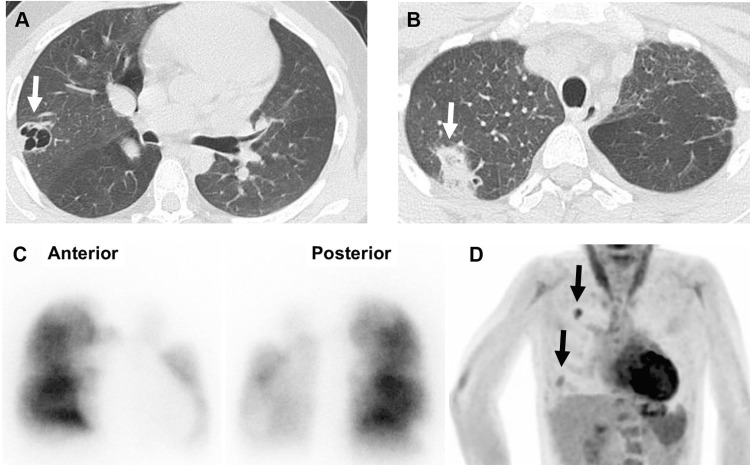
Images at admission A: Computed tomography (CT) indicated vesicles in the right lung (arrow), implying the possibility of pneumonia, although we needed to differentiate this from the other pulmonary diseases. This patient was negative for tuberculosis or fungal infection. B: Follow-up CT image after three months is shown. Although the original vesicle observed in panel A disappeared, other consolidation lesions with vacuoles were identified (arrow). C: Pulmonary blood flow scintigraphy showing marked disappearance of left lung perfusion. D: ^18^F-fluorodeoxyglucose-positron emission tomography revealed no signals in the heart or other organs, and only multiple hot lesions in the right lung were observed (arrows).
